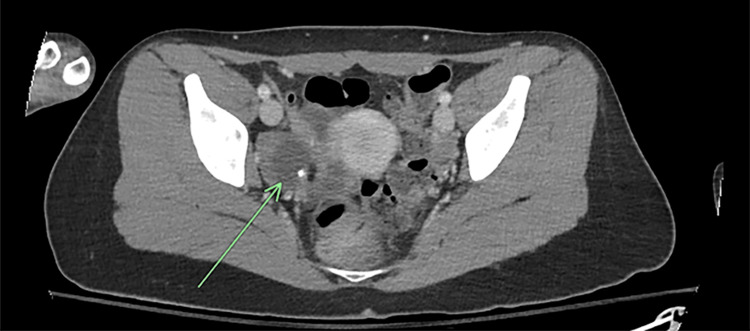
CT abdomen/pelvis, axial image. The arrow indicates 5.1 x 3.9 cm benign right adnexal teratoma.
